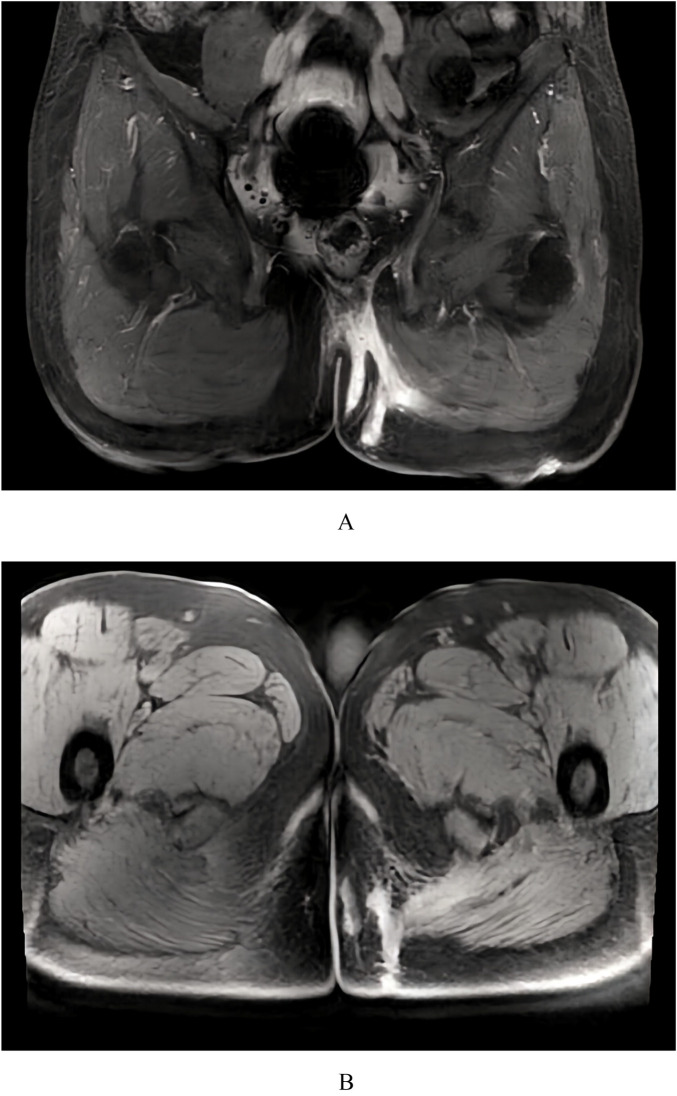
Pelvic contrast-enhanced MRI. Coronal (A) and axial (B) views demonstrating enhancing sinus tracts extending from the left gluteal region through the gluteus maximus muscle to the dorsal aspect of the left thigh root.
